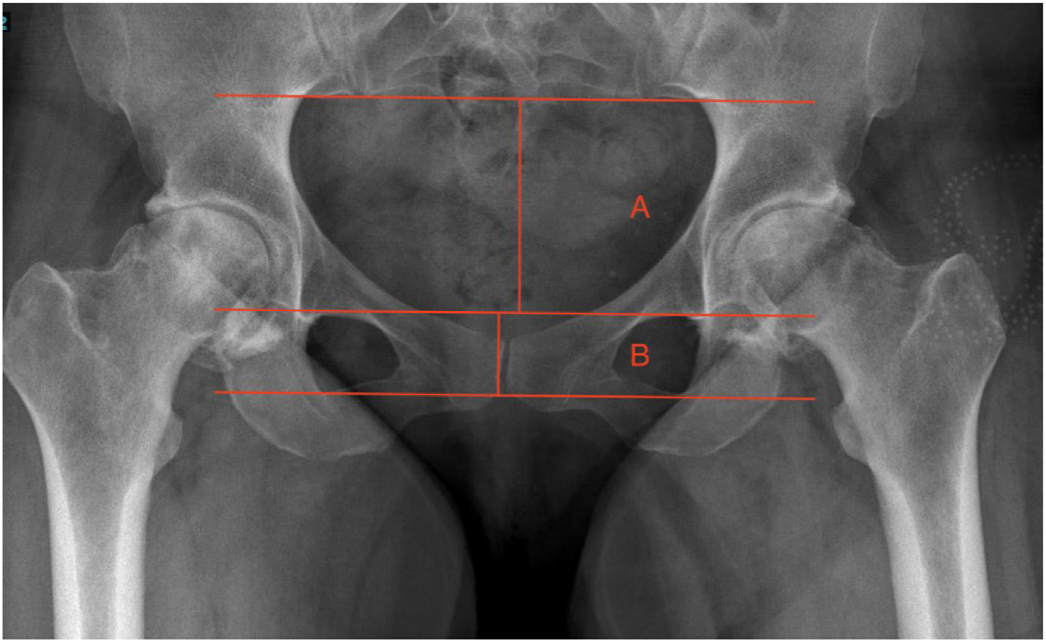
Preoperative AP plain film. Value A: Distance from the base of the sacroiliac joint to the superior margin of the obturator foramen. Value B: Height of the obturator foramen. Preoperative pelvic tilt was measured using the formula from Schwarz et al [18]: Tilt = −(ln[(B/A) × (1/0.483)]) / 0.051.
